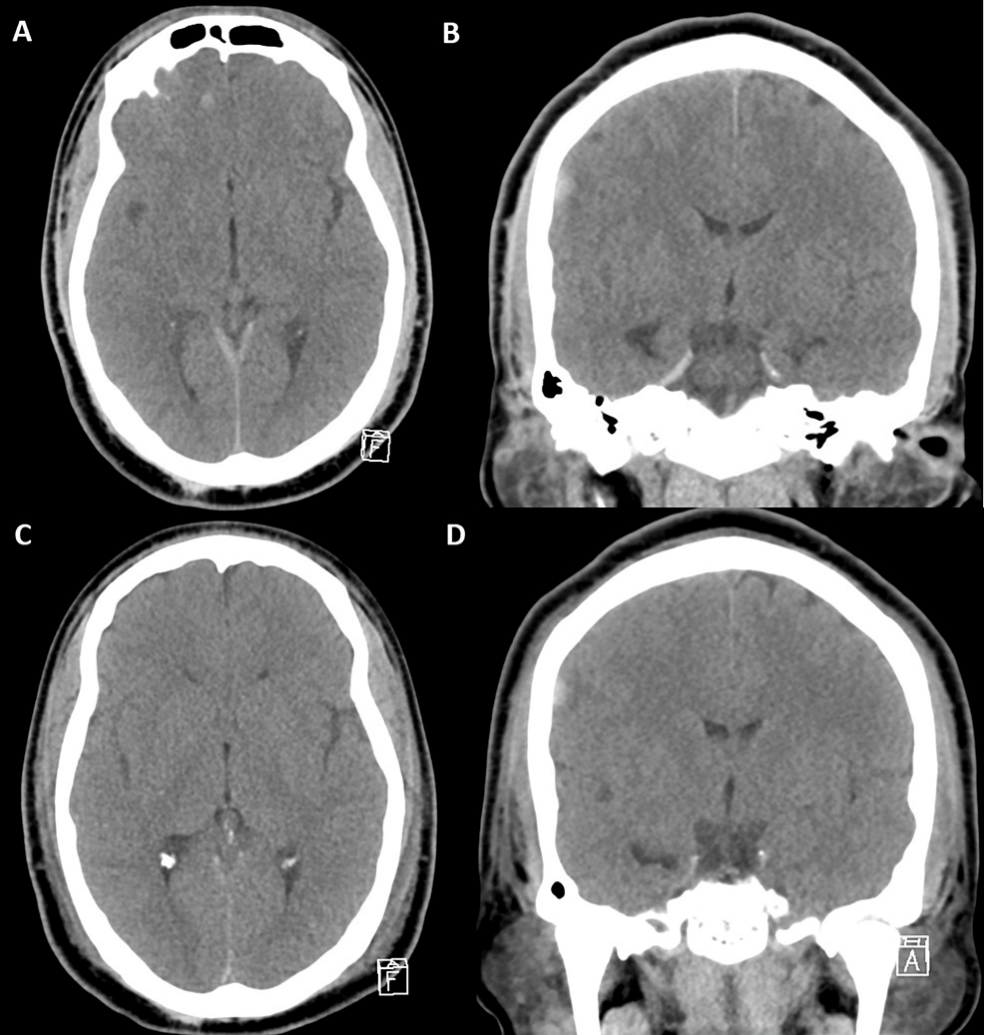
Axial and coronal slices of computed tomography scans of the head demonstrating a small right convexity subdural hematoma without herniation before orthopedic fixation of fractures (A and B), which remained stable postoperatively (C and D).
